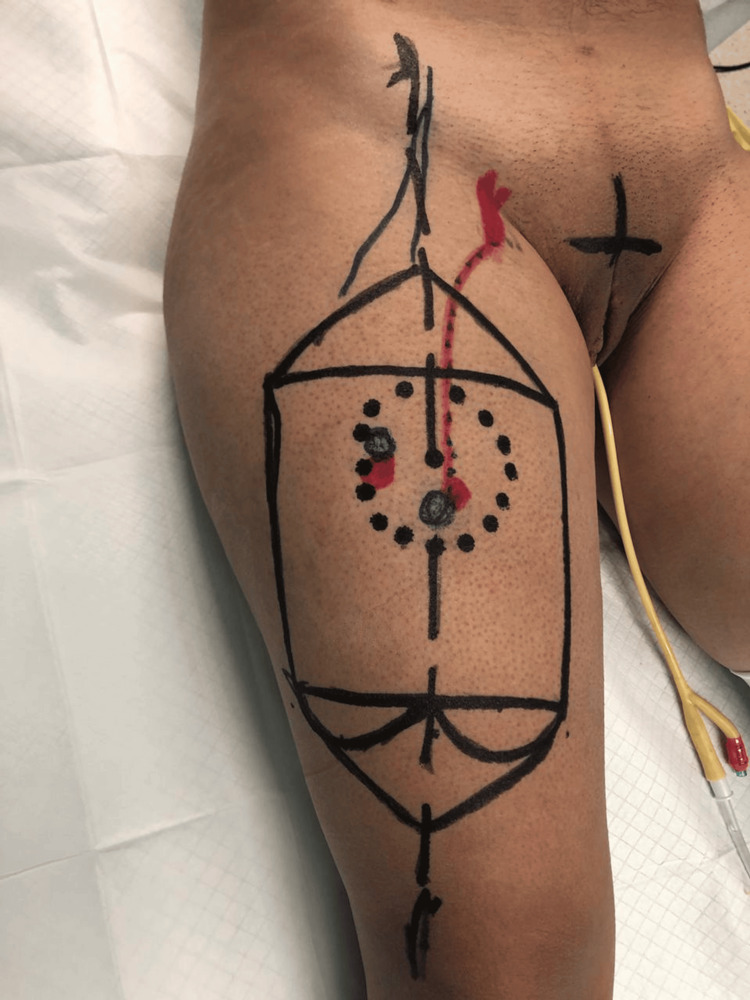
Design of the anterolateral thigh (ALT) flap and marking of the perforating vessels using Doppler sonography. The four large dots, located near the dotted line, depict the perforator vessels of the flap. The rectangle with the triangles at the top and the bottom represents the future phallus.
